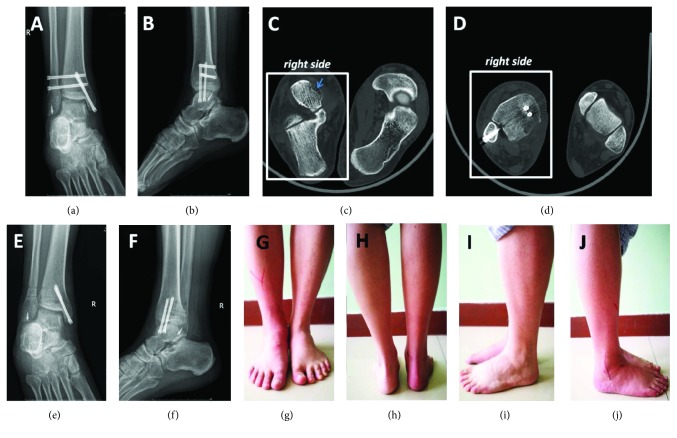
3 months posttreatment: anteroposterior and lateral radiograph images, radiographs after the removal of the syndesmosis screw fixation images, CT scanning of the ankle, and images of the patient standing on his feet. (a) Anteroposterior radiograph of the right ankle. (b) Lateral radiograph of the right ankle. (c) The astragalus contusion is indicated with the arrow. (d) The Dupuytren fracture and dislocation was ameliorated. (e) Anteroposterior radiograph of the right ankle after the removal of the syndesmosis screw fixation images. (f) Lateral radiograph of the right ankle after the removal of the syndesmosis screw fixation images. Images of the patient standing on his feet. (g) Anteroposterior image of the patient standing on his feet. (h) Posteroanterior image of the patient standing on his feet. (i) Lateral image from the left of the patient standing on his feet. (j) Lateral image from the right of the patient standing on his feet.
